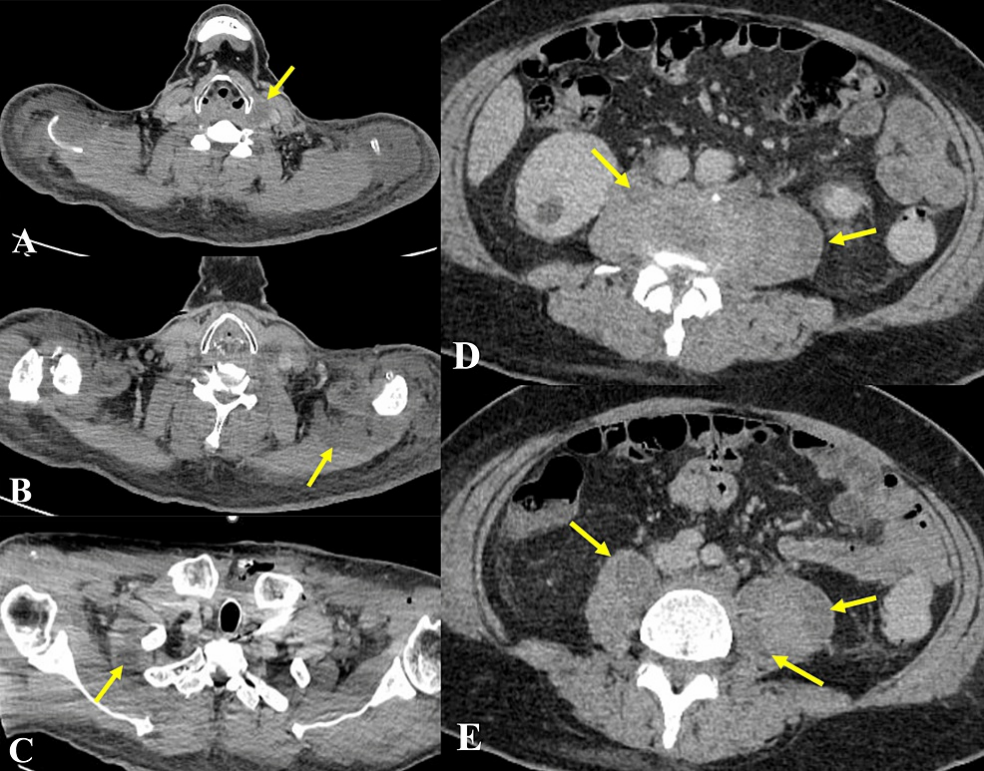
CT taken on hospitalization. Multiple abscesses located on the left side of the thyroid gland (A), ventral to the left trapezius (B), in the right serratus anterior (C), and in the bilateral psoas majors (D, E).
